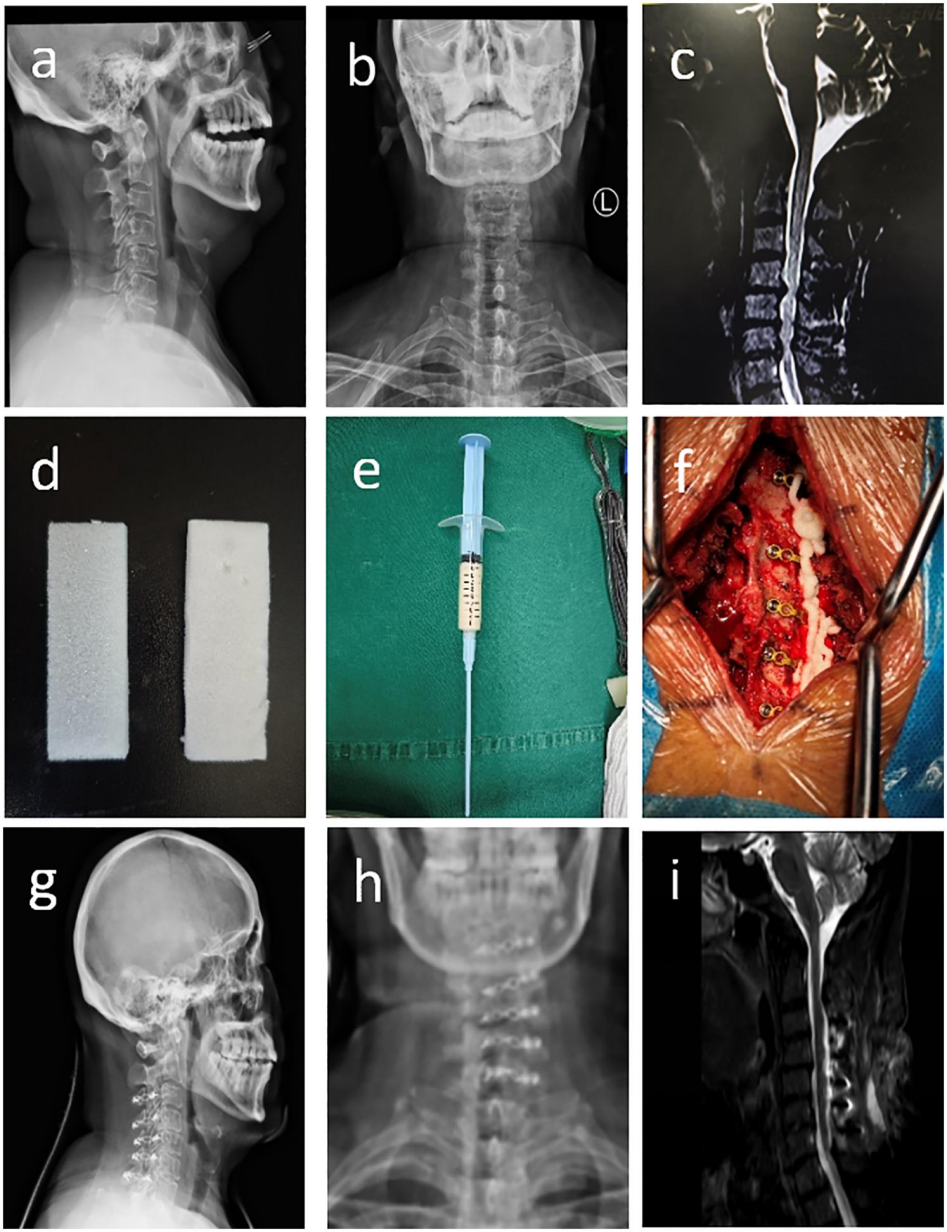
(a–c) Preoperative cervical spine radiographs (anteroposterior and lateral views) and MRI demonstrating C4-C7 disc herniations with spinal cord compressed and deformed; (d–f) absorbable gelatin sponge (GS), pre-mixed flowable gelatin hemostatic agent (HFG), and intraoperative application of flowable gelatin for hemostasis; (g–i) postoperative cervical spine radiographs (anteroposterior and lateral views) showing well-positioned internal fixation, and MRI demonstrating decreased spinal cord compression.
